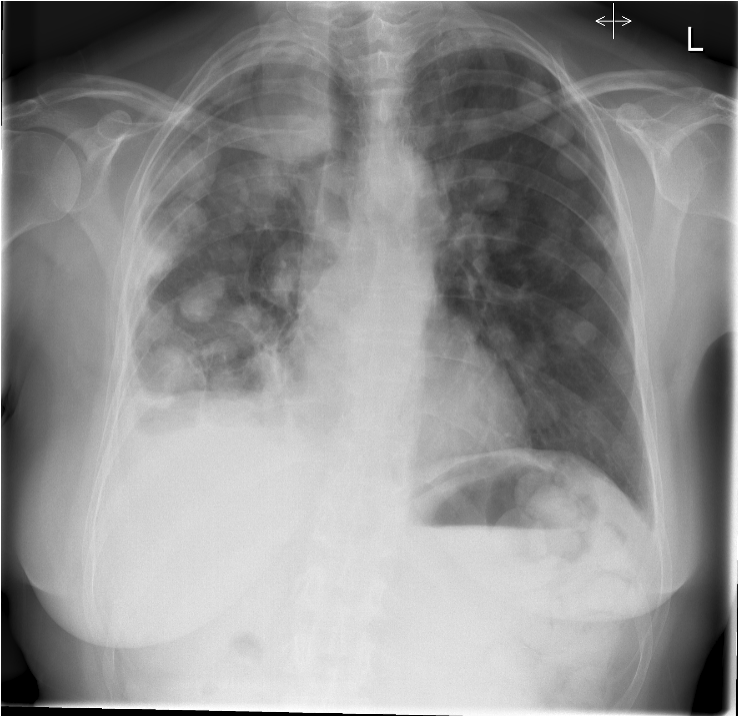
Distant metastasis-PA chest X-ray showing extensive cannon ball metastasis of the lungs.
